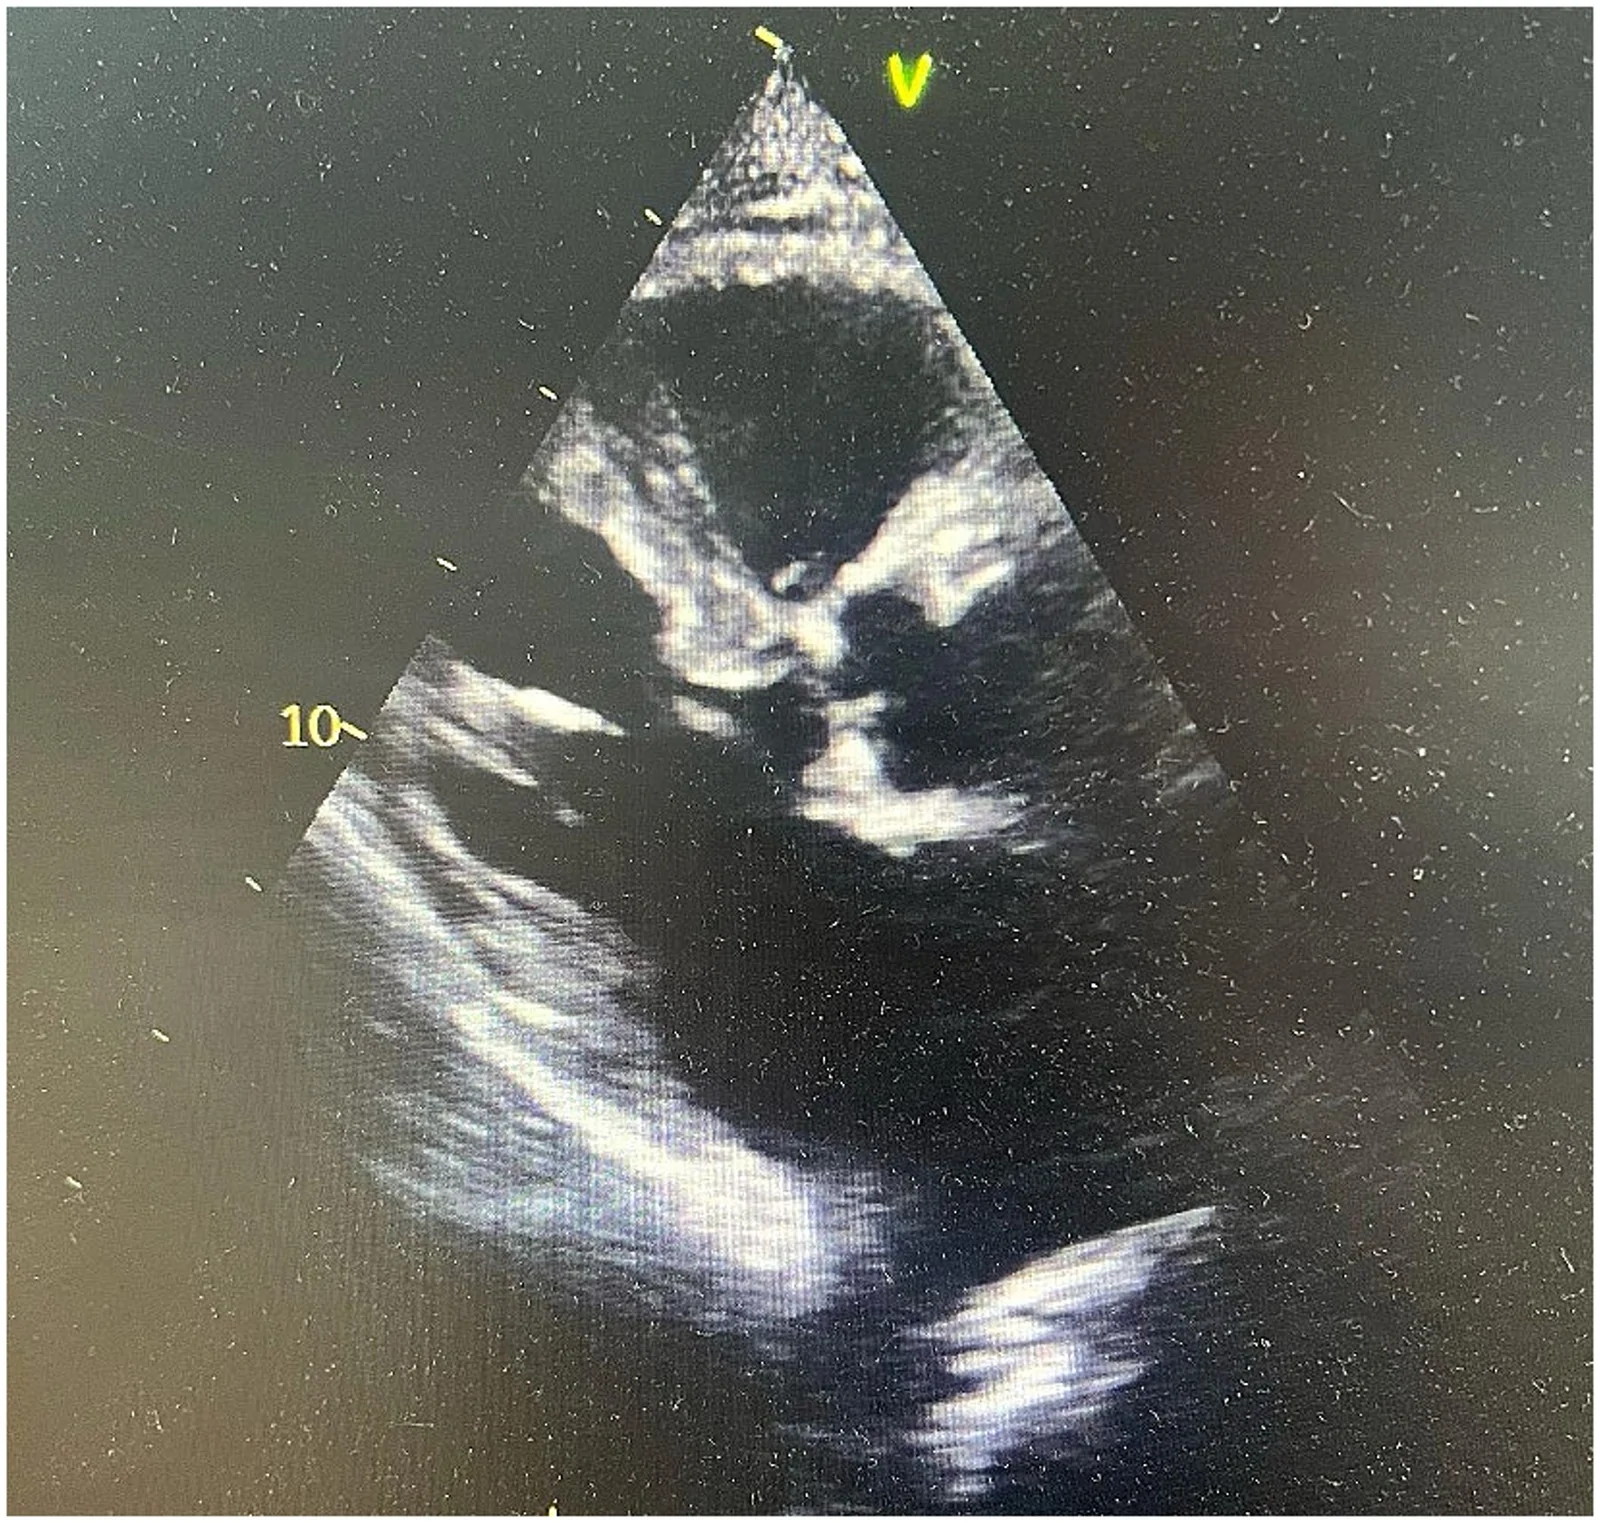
Pre-operative apical transthoracic echocardiographic view demonstrating severe aortic stenosis with markedly impaired left ventricular systolic function (left ventricular ejection fraction 15%).
